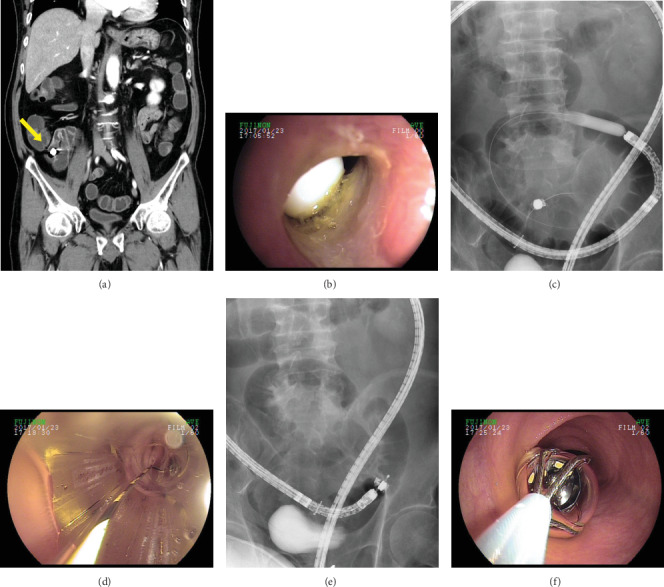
Successful retrieval of a capsule endoscope (CE) using a basket. The CE was located at the ileum on the abdominopelvic computed tomography image (yellow arrow) (a). Fluoroscopy-guided double-balloon endoscopy (DBE) showing that the CE was impacted by stenosing ulceration associated with nonsteroidal anti-inflammatory drug use approximately 60 cm proximal to the ileocecal valve (b). Enteroscopic balloon dilation was performed using a through-the-scope balloon dilation catheter (CRE™ balloon catheter; Boston Scientific, Natick, MA, USA) (c, d). After successful DBE-assisted balloon dilation, the capsule endoscope was retrieved using a basket (e, f).
